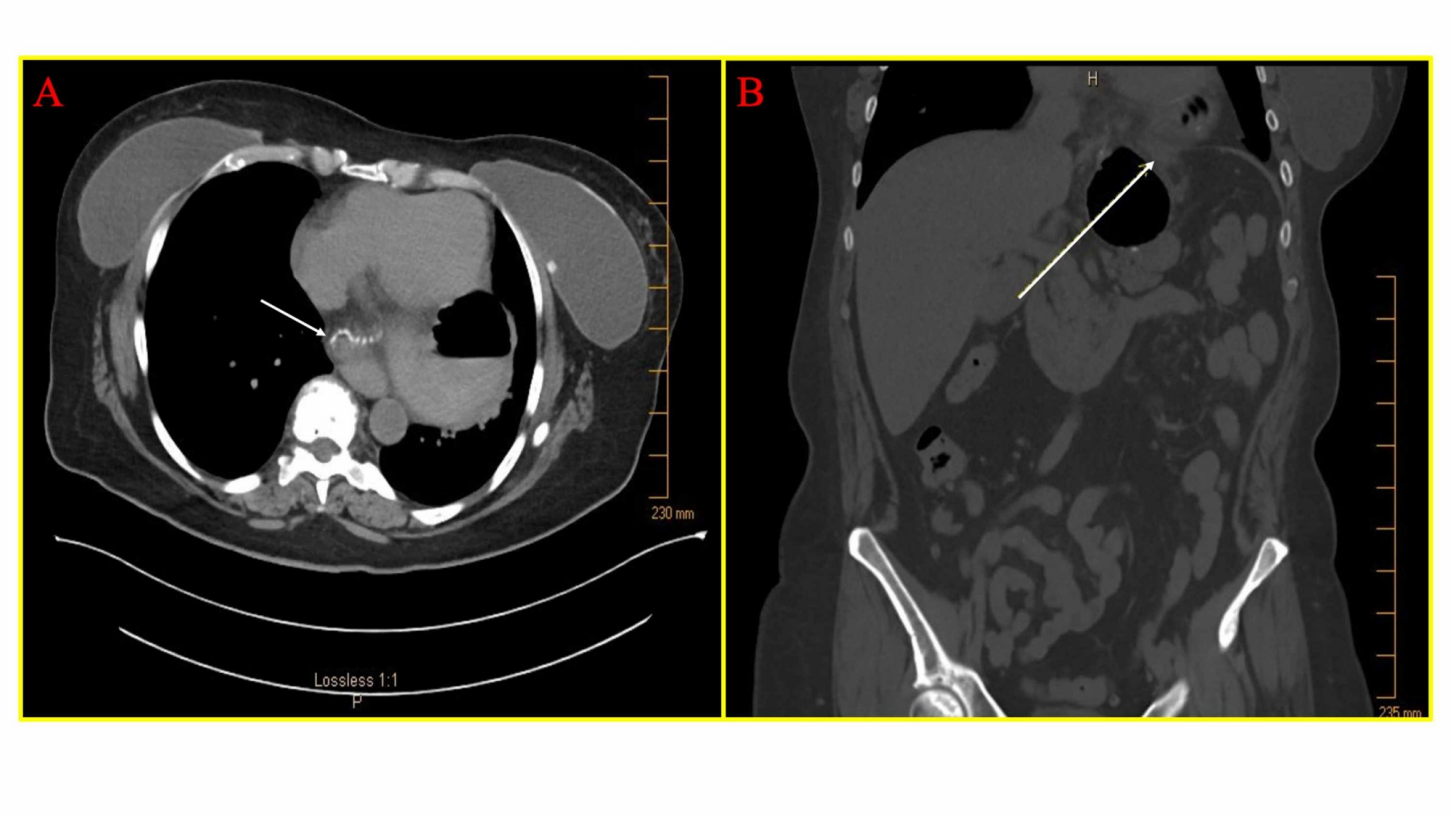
Pre-operative imaging demonstrating gastric pouch and proximal Roux-limb herniation Computed tomography abdomen and pelvis without contrast showing (A) axial and (B) coronal sections demonstrating the herniated Roux limb and gastric pouch prior to surgery (white arrows, herniated Roux limb).
